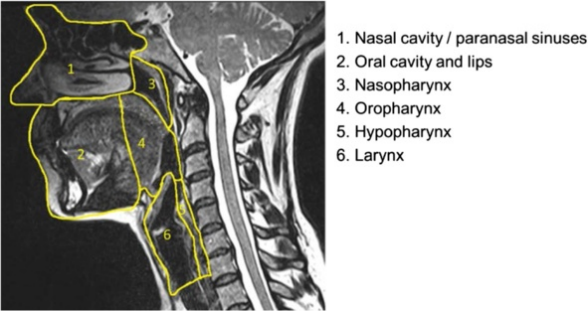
Sagittal T2-weighted image of the extracranial head and neck showing the division of the anatomical and functional units of the upper aerodigestive tract
